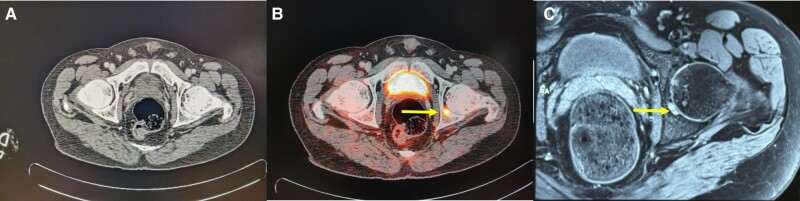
A, Plain computed tomography (CT) scan showing no lesion. B, Ga-68 DOTANOC PET/CT image showing tracer uptake involving posterior pillar of the left acetabulum with the lesion protruding intra-articularly. C, Magnetic resonance imaging scan showing a 3.5- ×1.5-mm lesion involving the posterior column of the left acetabulum and extending into the subchondral bone posterior to the fovea of the femoral head.
